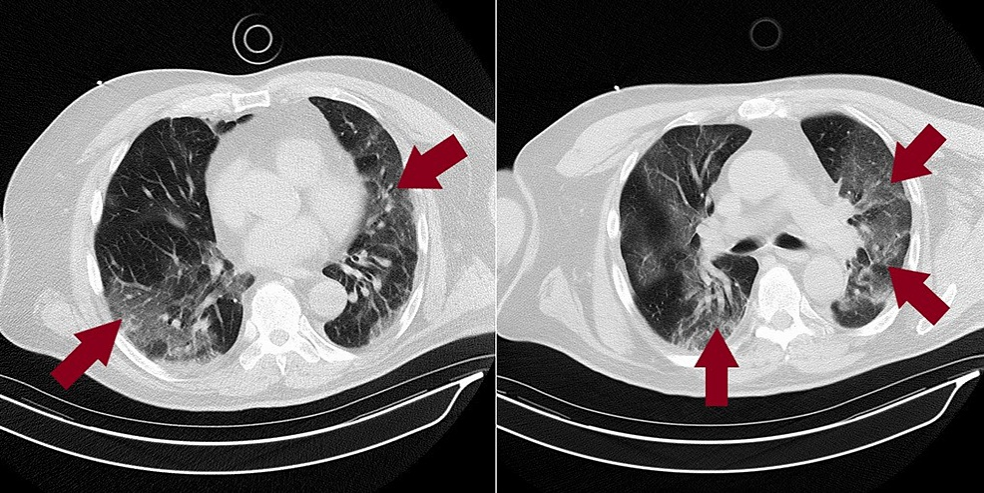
CT chest without contrast Bilateral diffuse nonzonal predominant airspace opacifications in the lung parenchyma consistent with manifestations of atypical pneumonia (maroon arrows) CT – computed tomography
